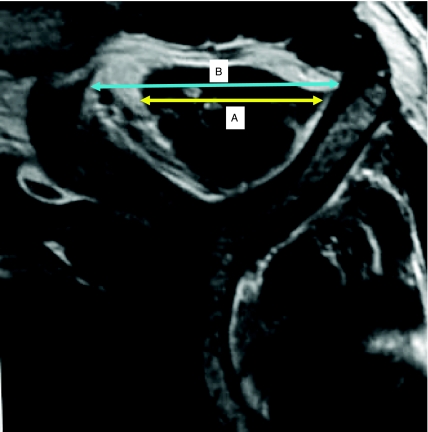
Muscle atrophy measured by the cross-sectional area of the supraspinatus fossa on sagittal oblique MRI. The occupancy ratio of the supraspinatus A/B × 100 (%). A = maximum width of the supraspinatus. B = maximum width of the supraspinatus fossa.
